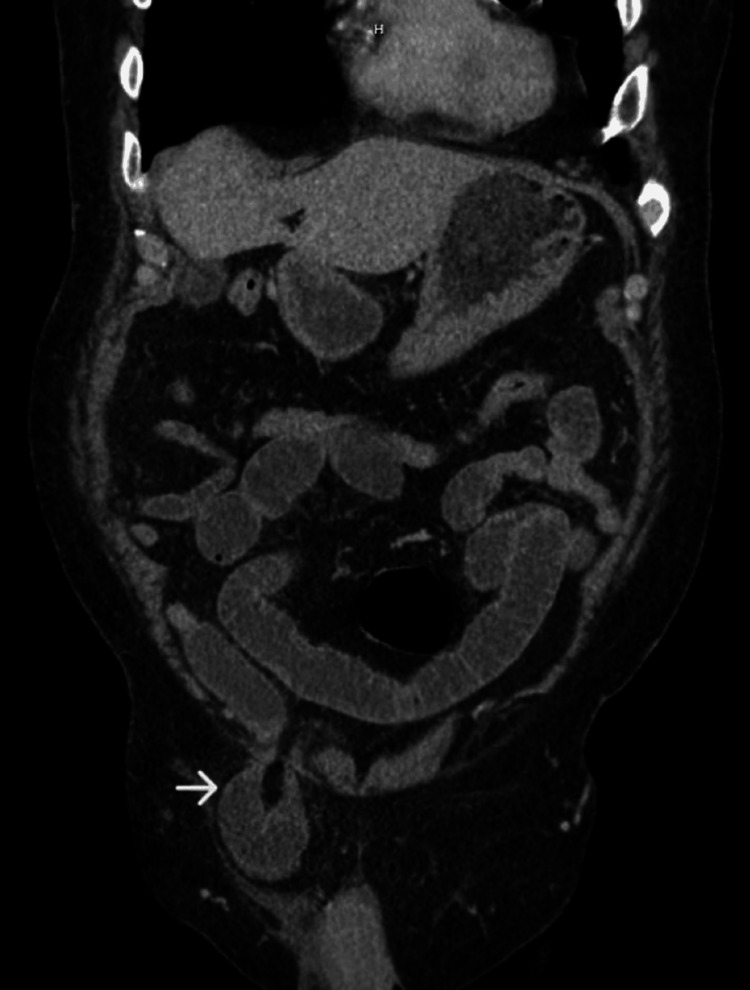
A CT scan image showing a right inguinal hernia containing a bowel loop (→)
